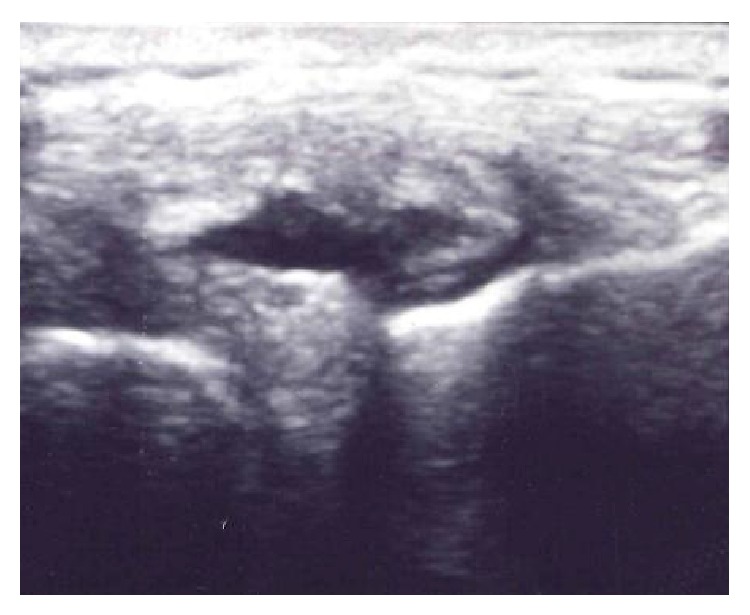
Ultrasound image in the coronal plane demonstrating an anechoic meniscal cyst (arrow) superficial to the lateral meniscus.
